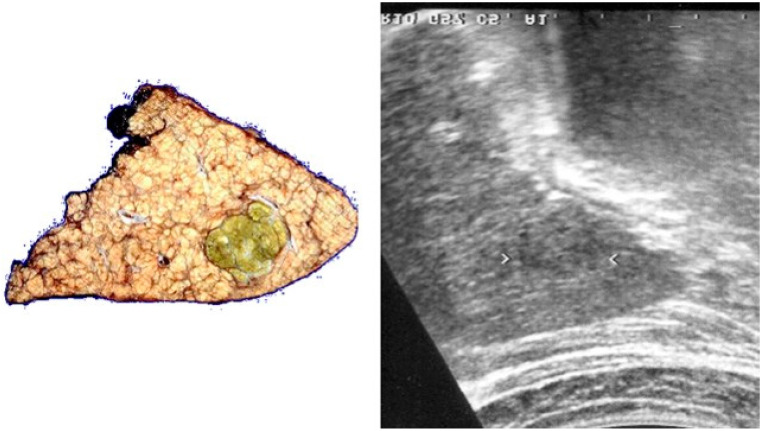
Example of very early HCC in the resected left lobe of the liver. The perfect correlation between the pathological finding (on the left) and the sonographic appearance of the very early HCC nodule (arrowheads) (on the right) is observed.
